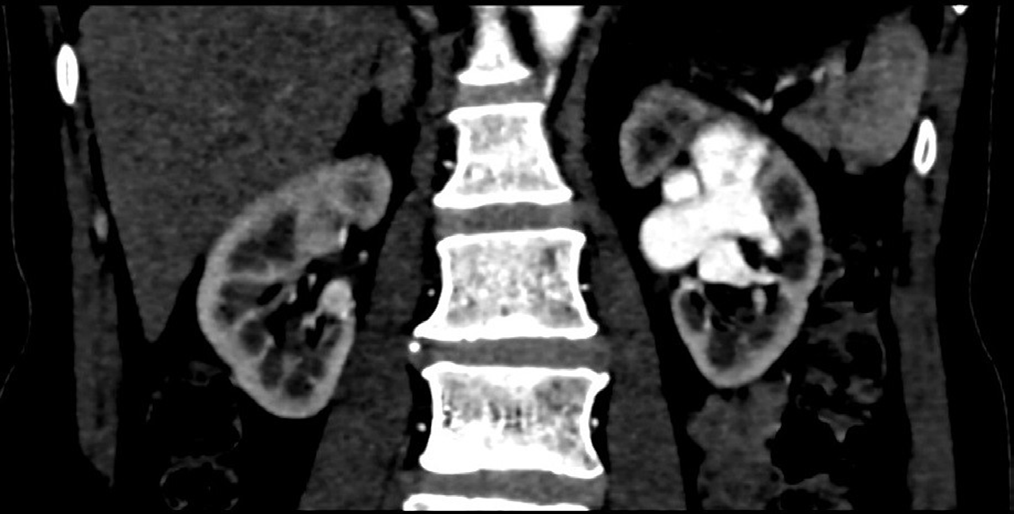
Contrast CT Urogram (arterial phase-coronal). At least two direct fistula
